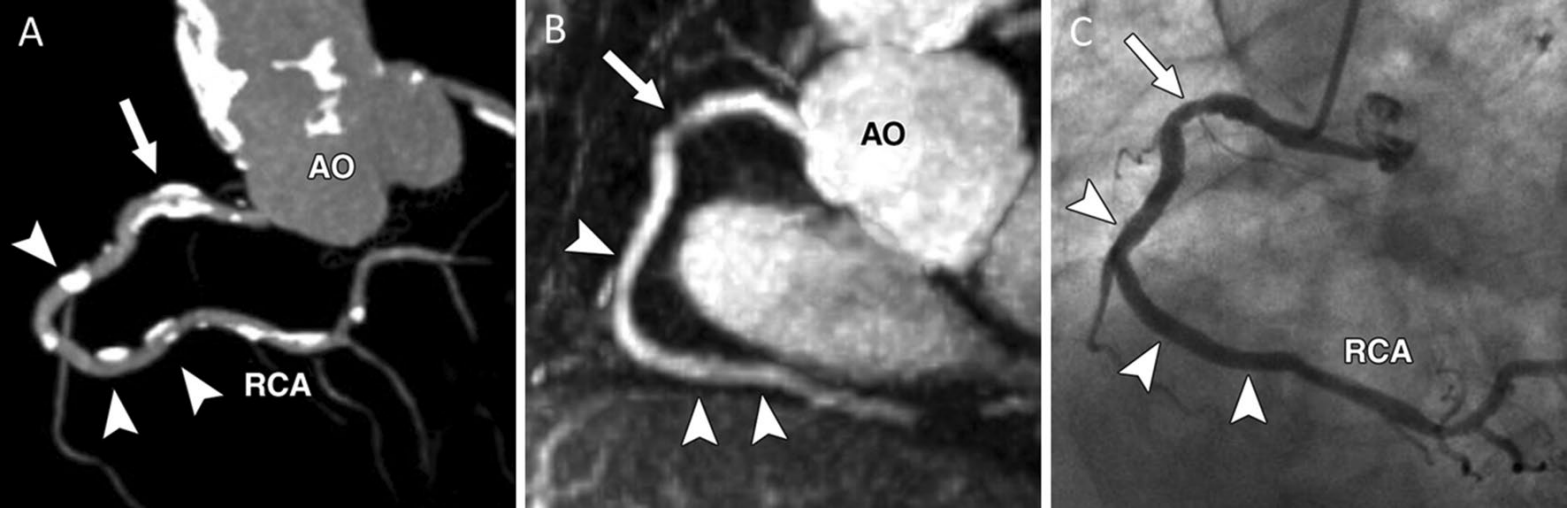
Representative images of the RCA in three different modalities of a coronary CTA, b coronary MRA, and c CAG. a Diffuse calcification (arrow and arrowheads) was detected in RCA on coronary CTA MIP image. Coronary MRA MIP image b shows moderate stenosis (arrow, b) and CAG (c) confirms moderate stenosis (arrow, c) in corresponding segment where heavy diffuse calcification can be seen in a (arrow in a). b, c No significant stenosis (arrowheads) in corresponding segments where nodal calcifications are located in (a). *AO* aorta, *RCA* right coronary artery, *CTA* computer tomography angiography, *MRA* magnetic resonance angiography, *CAG* coronary angiography, *MIP* maximum intensity projection. (Reprinted with permission from Liu et al. [37], Copyright © 2007 by the American Roentgen Ray Society, ARRS)
